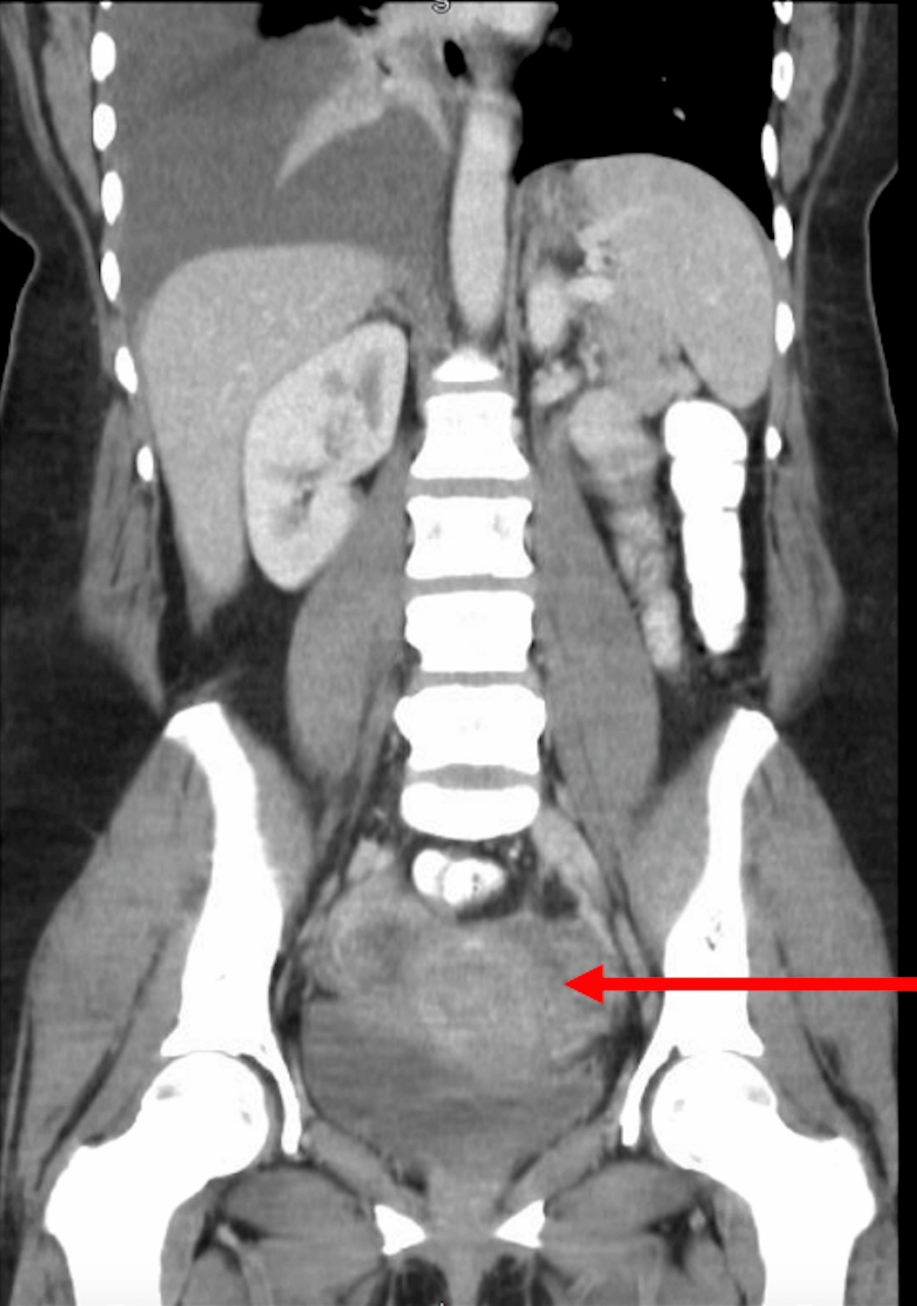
Coronal View of CT Abdomen/Pelvis Without Contrast Showing a Mixed Solid and Cystic Mass Within the Left Ovary Red arrow highlighting the area of interest. CT: computed tomography
